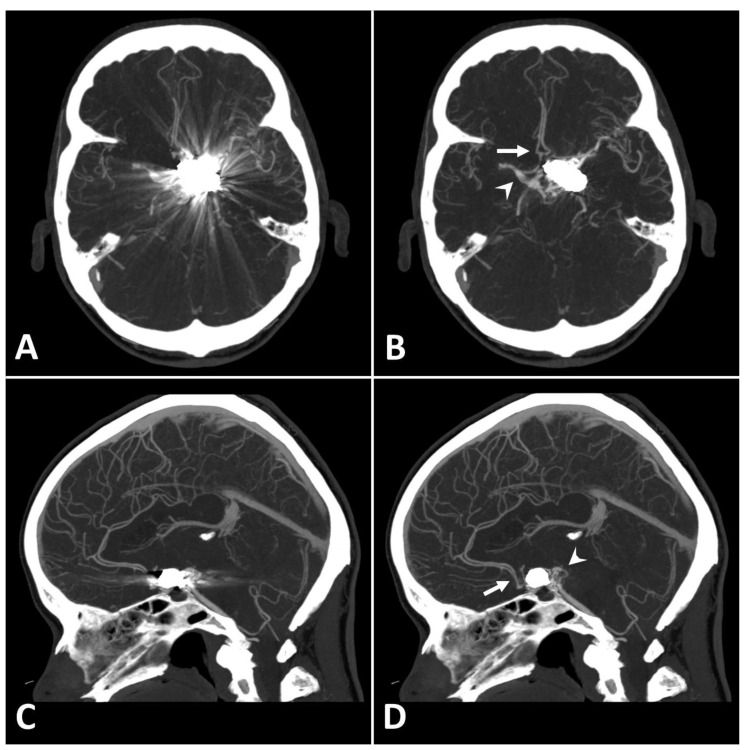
Exemplary follow up UHR-CT-angiography in axial (A,B) and sagittal (C,D) plane without (A,C) and with SEMAR (B,D) of a 56-year-old female after intracranial coil-embolization of a left sided posterior communicating artery aneurysm. All images were reconstructed as MIP (Maximum Intensity Projection) with 12.5 mm slice thickness. SEMAR significantly improves image quality, even in vessels directly adjacent to the coil package like the anterior communicating artery (white arrow in (B)), the contralateral terminal carotid artery (arrowhead in (B)), the anterior cerebral artery (white arrow in (D)), and the posterior communicating artery (arrowhead in (D)).
